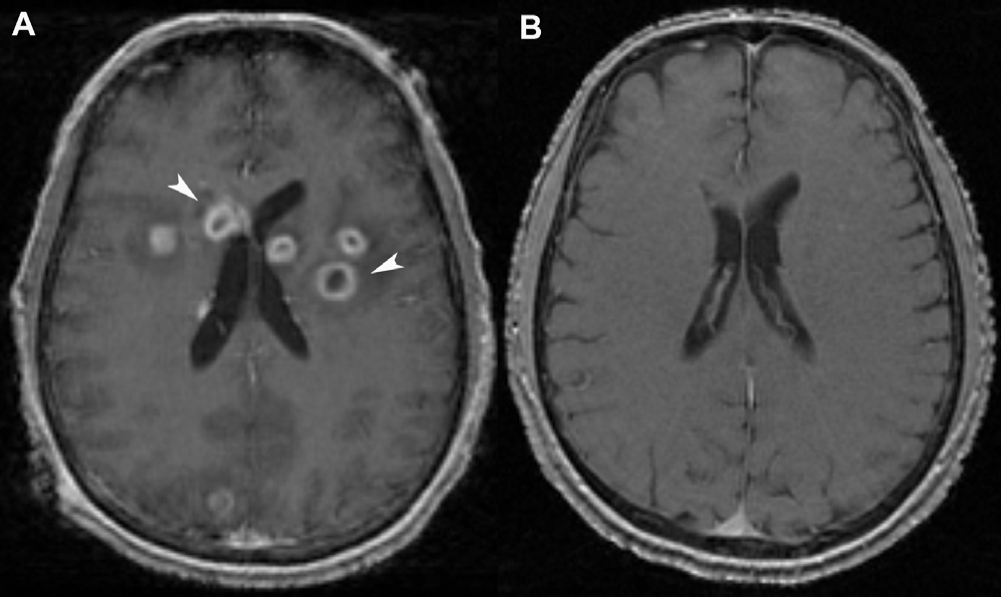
Magnetic resonance imaging of the brain. (A) T1-weighted images at the patient's presentation, post-contrast showing multiple ring enhancing lesions in the brain (white arrow heads). (B) T1-weighted images eight months after treatment showing a resolution of the brain abscesses.
